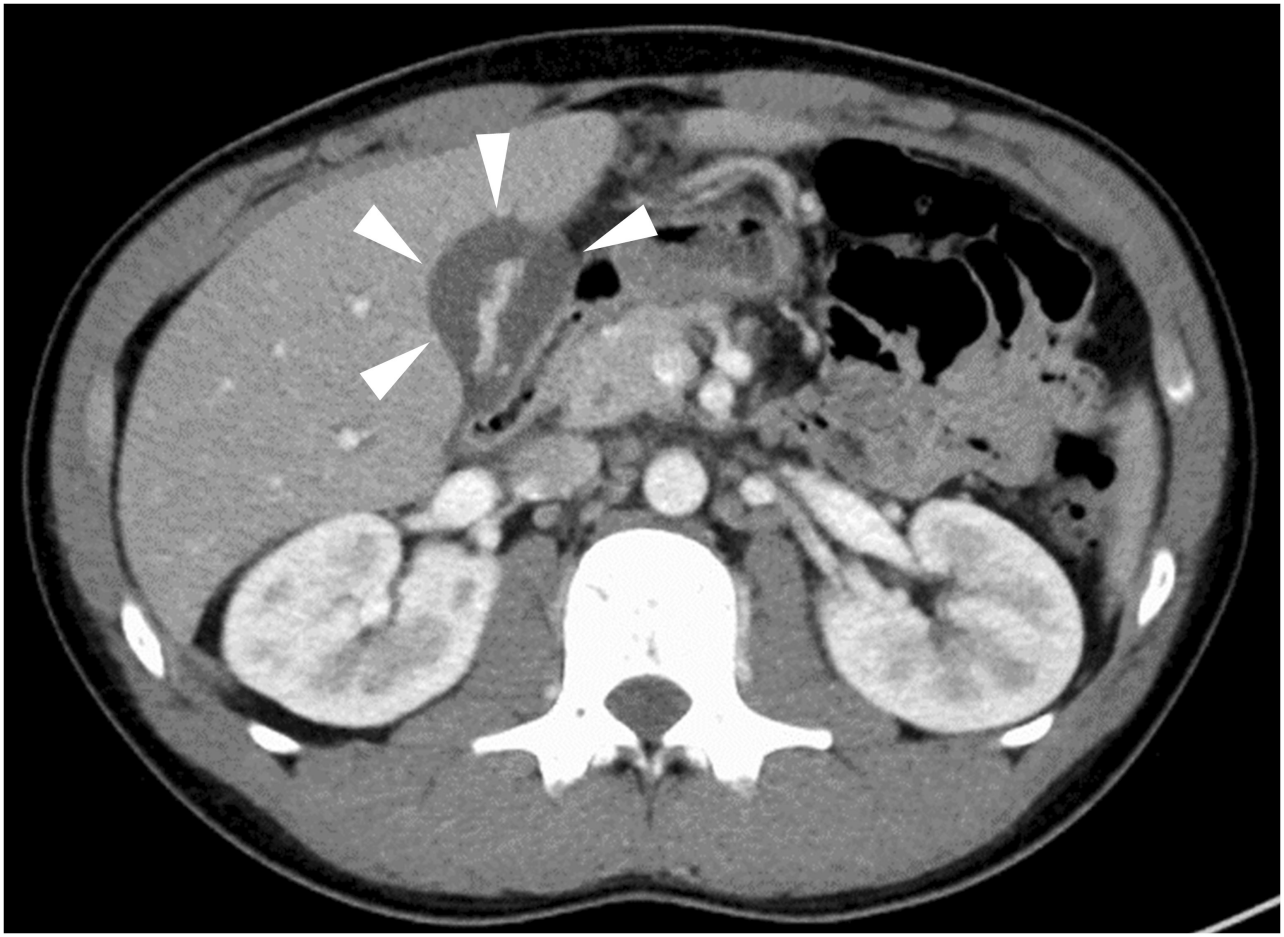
Abdominal contrast‐enhanced CT (axial view). Edematous wall thickening is shown (arrowheads).
